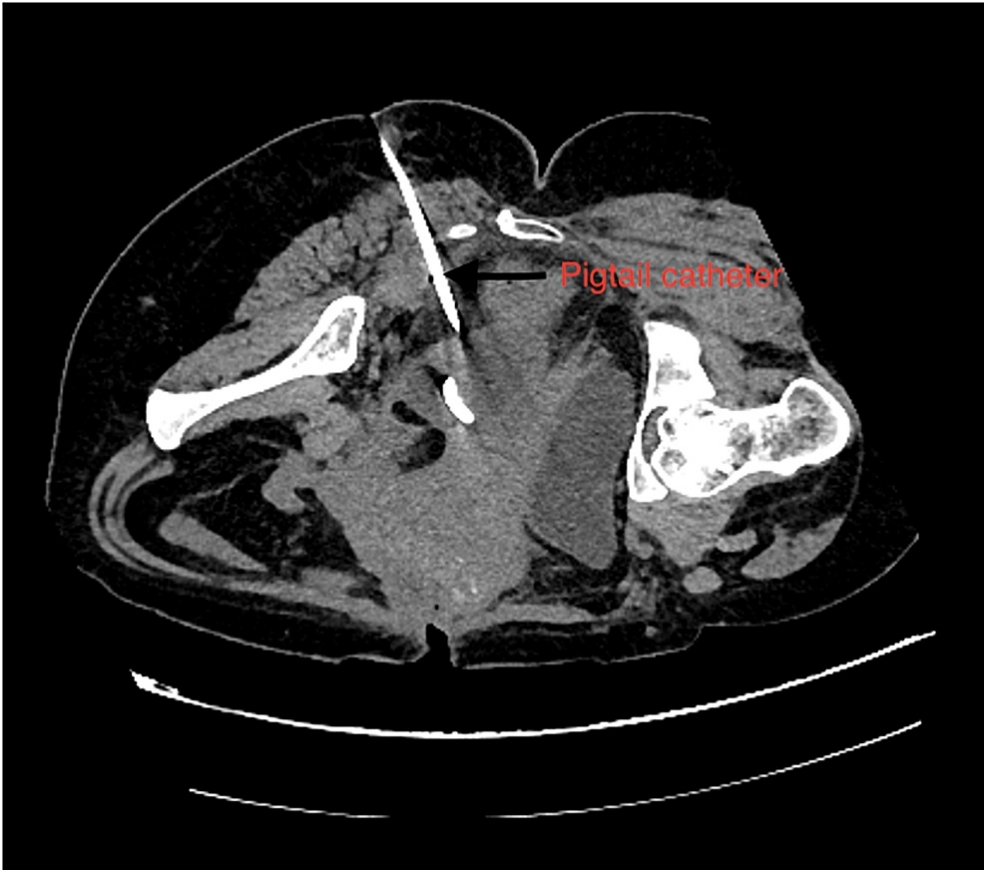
CT Scan image showing insertion of pigtail catheter after needle placement via transgluteal approach.
